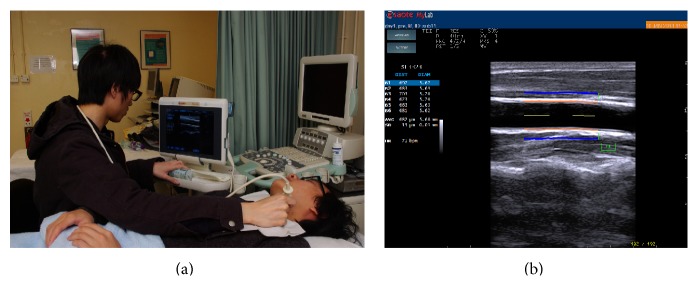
Ultrasound Scanning of the Carotid Artery*. The setup of the ultrasound measurement of cPWV at a site 1 cm proximal to the bifurcation of the left common carotid artery (a). The measurement interface of RFQAS program (b).
